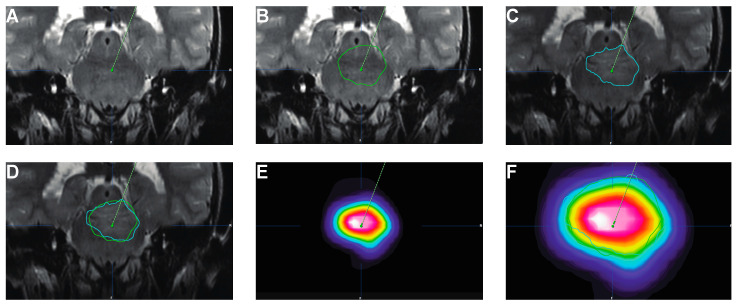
Examples of Predictive software for Vd. T2-weighted MRIs showing (A) actual catheter positioning prior to infusion; (B) predicted Vd before infusion (green outline; 9.8 mL); (C) actual Vd following infusion determined as ΔT2 (blue outline; 9.6 mL); (D) overlap between predicted (green) and actual (blue) Vd. (E) PET image showing actual tracer distribution following delivery. (F) Magnified PET image showing actual tracer distribution following delivery projected over predicted Vd (green) and actual Vd (ΔT2, blue). Images courtesy of Eva Wembacher, BrainLab (Munich, Germany).
